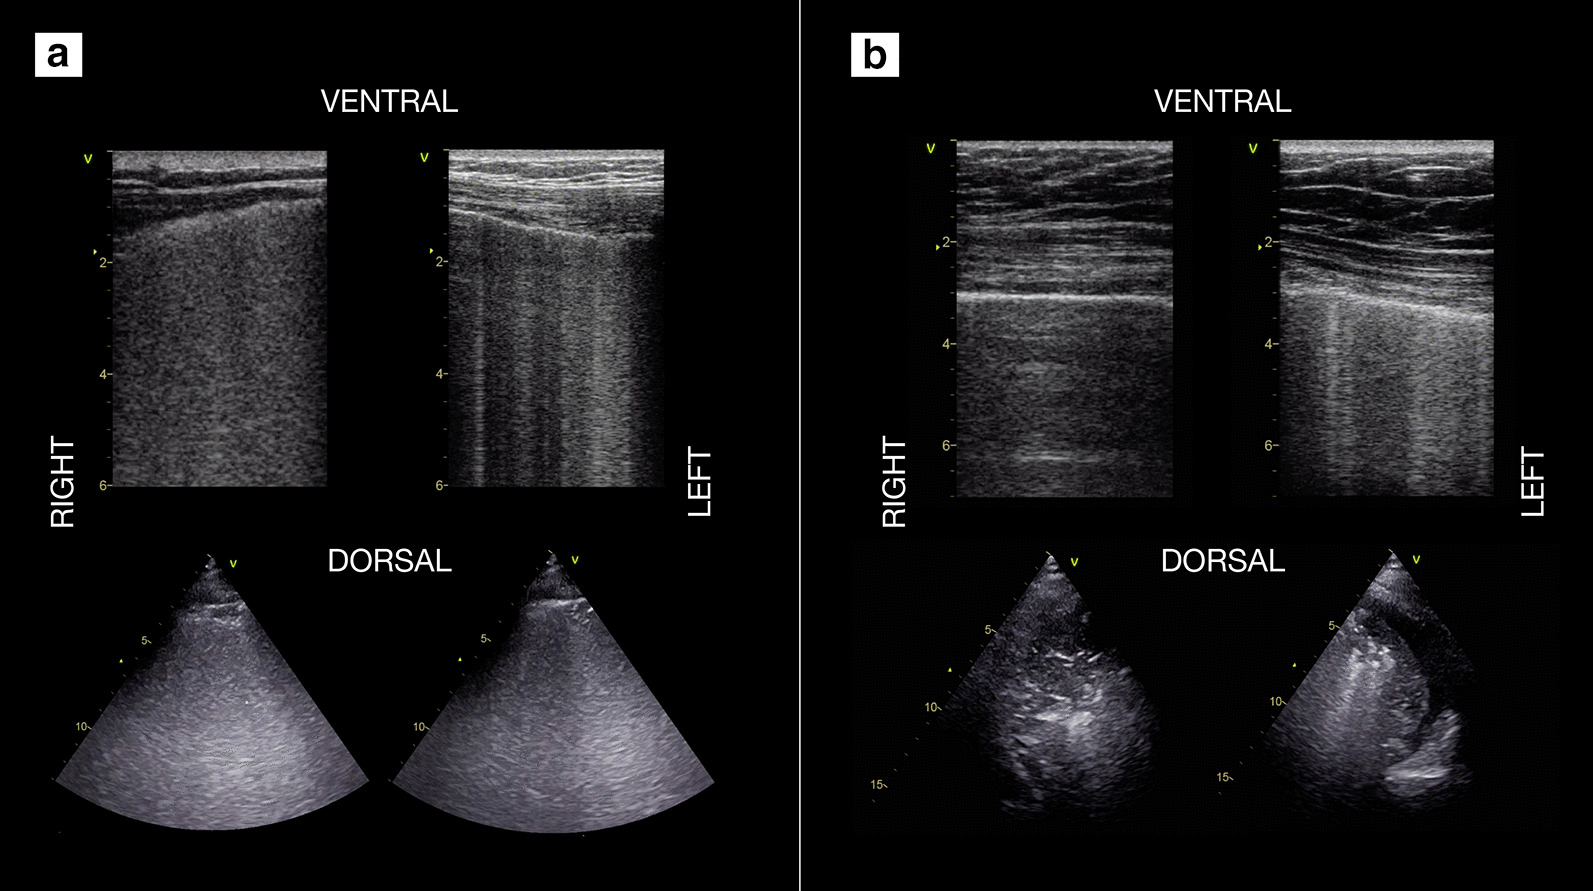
Use of lung ultrasound to monitor lung aeration and guide ventilatory management in 2 COVID-19 patients. a COVID-19 patient on day 2 after intubation and ICU admission, initially with PEEP 12 cmH_2_O: diffuse bilateral B-pattern with crowded, coalescent B-lines (“white lung appearance”) is visible, consistent with a sonographic interstitial syndrome and severe loss of aeration/increase of extravascular lung water. Based on these findings and on respiratory mechanics, a stepwise recruitment maneuver with a final PEEP set at 15 cmH_2_0 was performed, with improvement in gas exchange. b A different COVID-19 patient on day 4; PEEP set at 14 cmH_2_O: in comparison with previous patient, less B-lines are visible in ventral scans, with asymmetric distribution (more on the left scan); dorsal areas show lung consolidations, larger on the right side, with air bronchograms (dynamic at live scan). A pronation trial was successful, yielding immediate improvement in gas exchange and subsequent re-aeration of dorsal areas. (Ventral scans are taken with a linear, high frequency probe, dorsal ones with a phased array low-frequency one)
